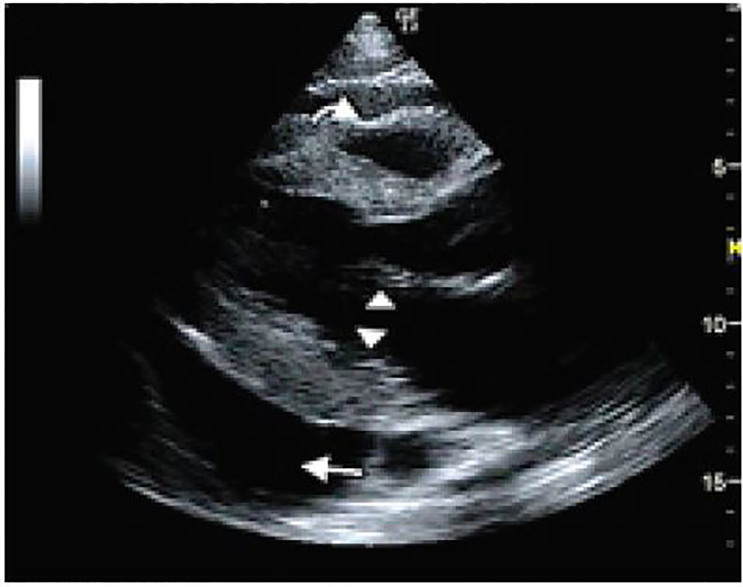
Parasternal long-axis view demonstrating a pericardial effusion (straight arrow) with right ventricular collapse (curved arrow) during diastole, as evidenced by opening of the mitral valves (arrowheads)
